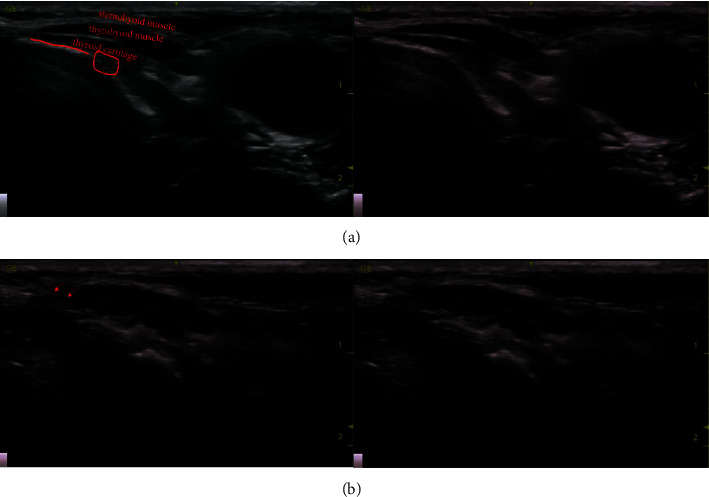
The figure shows ultrasound images. The red line in (a) shows the area of the thyrohyoid membrane, and the red circle in (a) shows the area of the internal branch of the superior laryngeal nerve and the superior laryngeal artery. The arrow in (b) shows the puncture needle.
